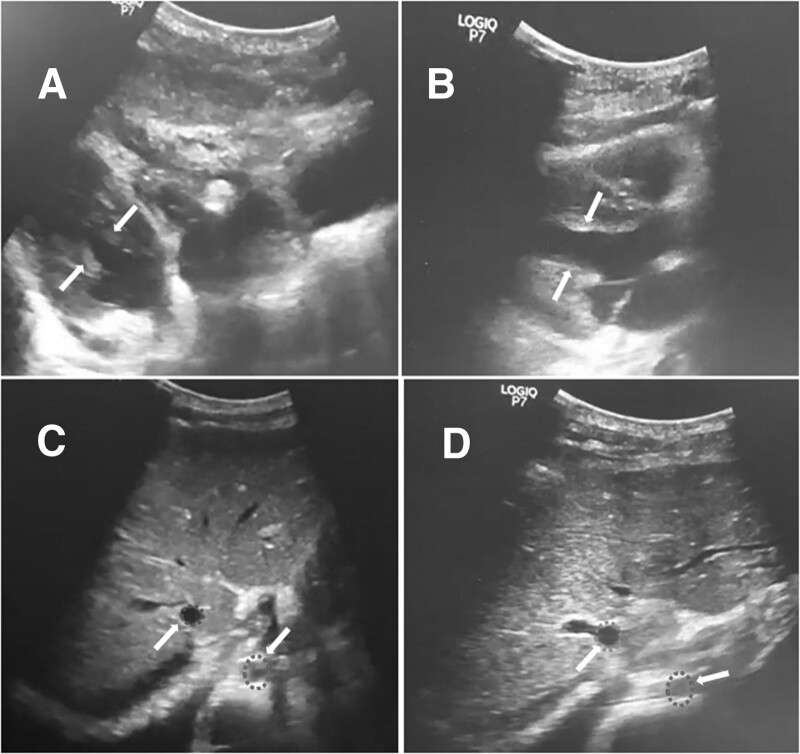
Point-of-care ultrasounds reveal mild pericardial effusion, significantly empty left and right ventricles, small end-diastolic volume of left ventricle (arrow lines), hyperdynamic heart and ejection fraction (EF) 50% (A), and substantial distention of the inferior vena cava (IVC) and IVC’s size (blue-colored dotted circle) closely half as the juxtaposed descending aorta (red-colored dotted circle) (C) at dengue obstructive shock. After shock management, there were significant improvements in early diastolic wall motion of the left ventricle, enhanced end-diastolic volume of left ventricle (arrow lines) and EF 65% (B), well distention and less variability of the IVC (D).
